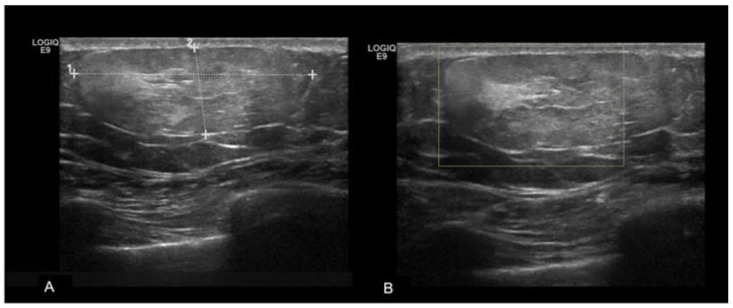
A 35-year-old men with a palpable lump in the inner quadrants of the left breast. On a B-mode (A) breast ultrasound, there is an oval shaped and hyperechoic mass, with circumscribed margins and parallel orientation. No posterior feature is associated. On color-doppler (B), there are no signs of vascularization. The imaging features are consistent with a lipoma.
